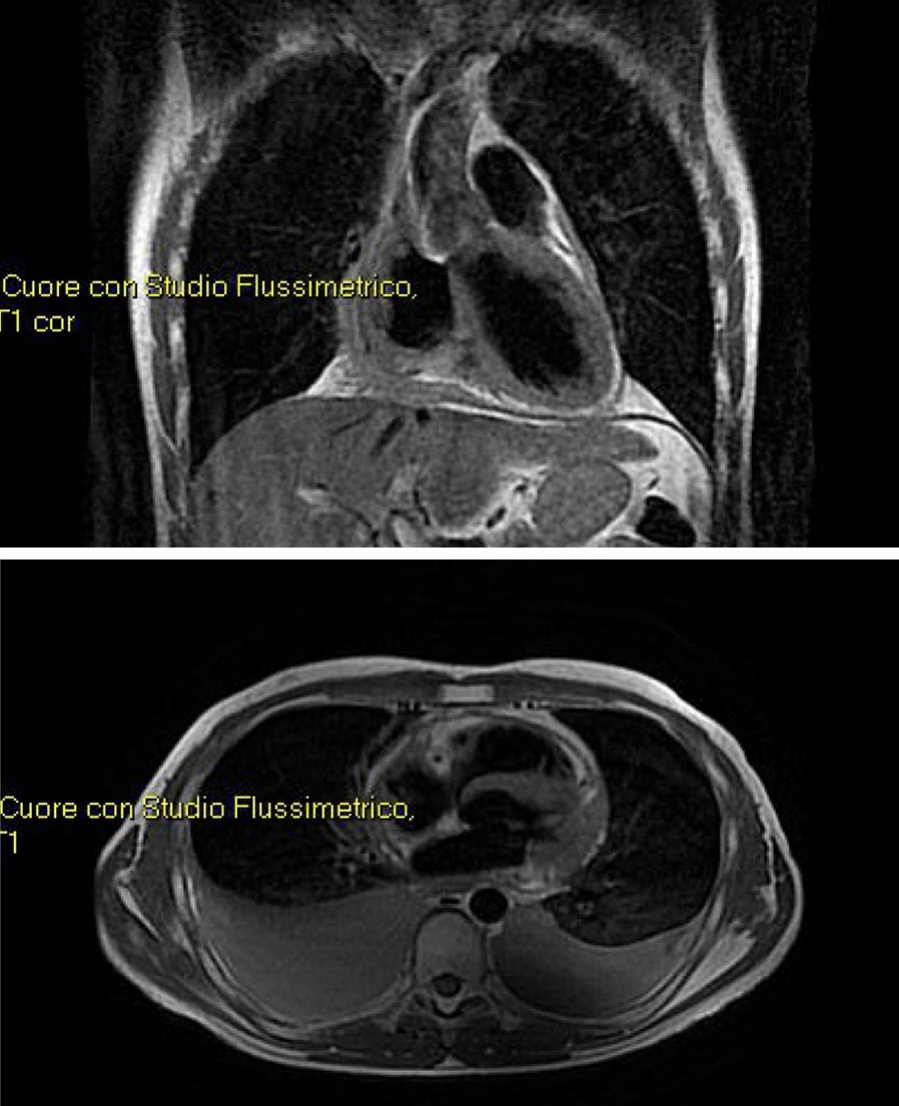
Nuclear magnetic resonance imaging after neoadjuvant therapy with docetaxel (24 administrations).
